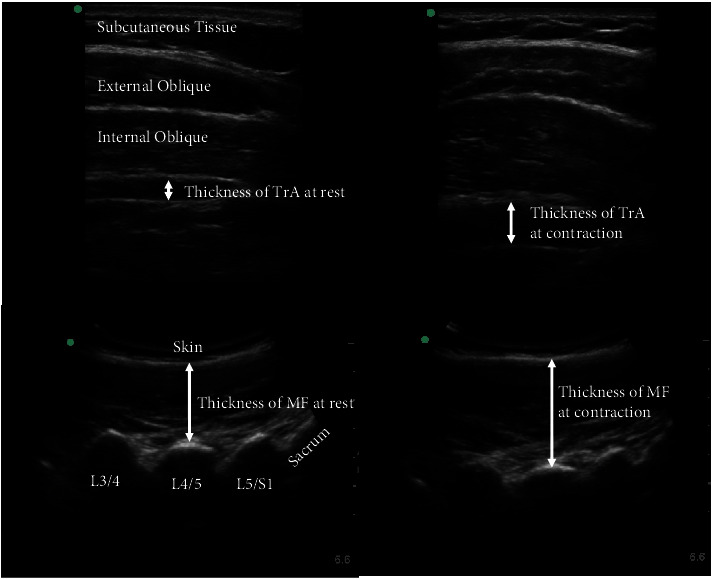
Ultrasound images and measures of MF and TrA. Abbreviations: MF, multifidus; TrA, transversus abdominis.
